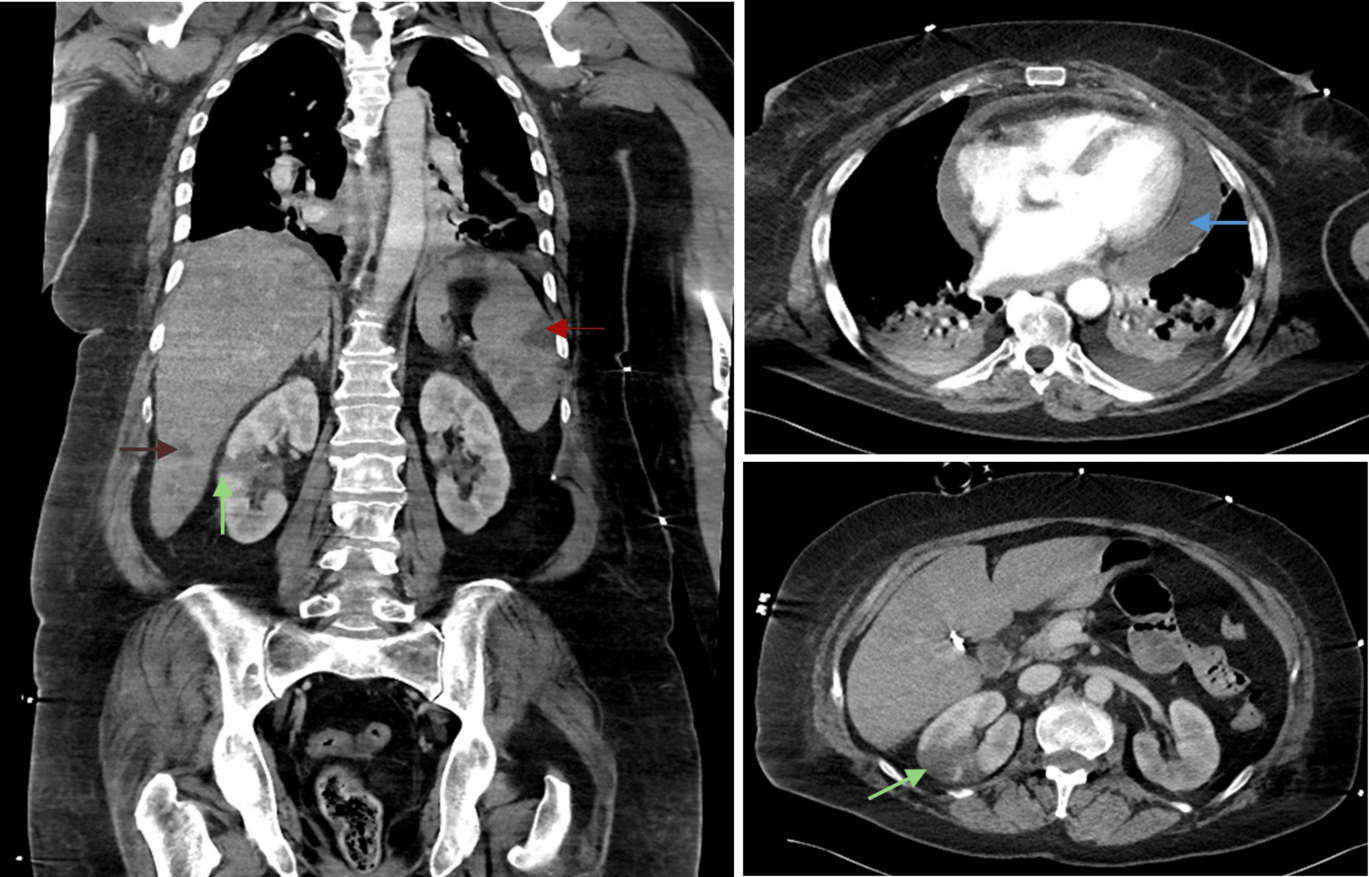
Complementary study during ICU stay - thoraco-abdominopelvic CT scan with angiography. Thoraco-abdominopelvic CT scan with angiography revealing high density pericardial effusion on the upper right (blue arrow), multiple renal (green arrows) and splenic (red arrow) infarction, and hepatic abscesses (brown arrow). ICU, intensive care unit; CT, computed tomography
